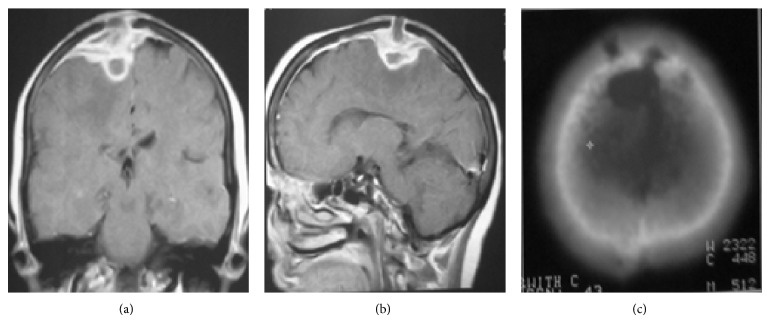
Tuberculous abscess with epidural and subdural empyema and calvarial osteomyelitis. Coronal (a) and sagittal (b) postcontrast T1-weighted MR images demonstrate epidural and subdural collections over the bifrontal cerebral convexities (more on the right side) with intraparenchymal and calvarial extension. Peripheral edema and irregular marked enhancement of the lesion as well as dural enhancement are evident. The bony destructive lytic lesions are seen in the bone window CT image (c).
